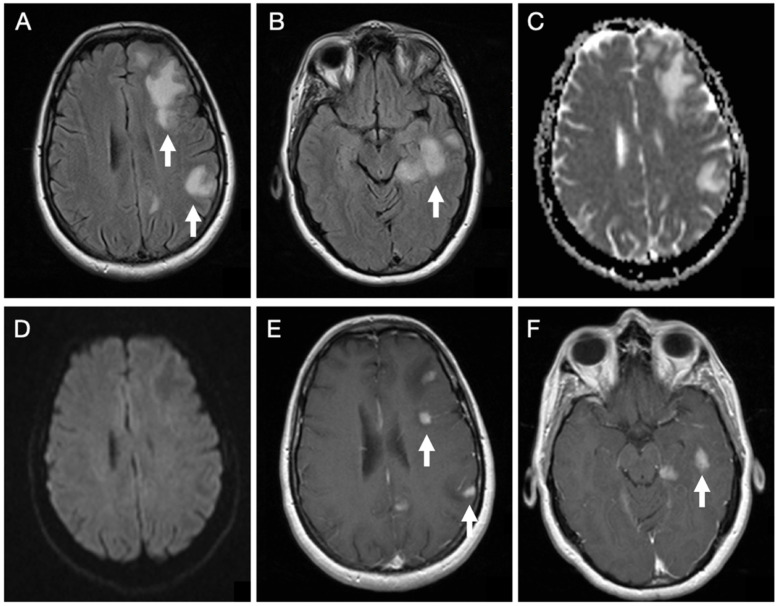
Initial brain MRI on axial FLAIR sequences of patient 1 demonstrates several hyperintense foci within the subcortical white matter of the left frontal, parietal, and temporal lobes (A,B). Diffusion-weighted imaging in the B1000 sequence and the corresponding ADC map reveal facilitated diffusivity of the lesions (C,D). Post-contrast T1-weighted images show multiple nodular enhancing foci (E,F).
